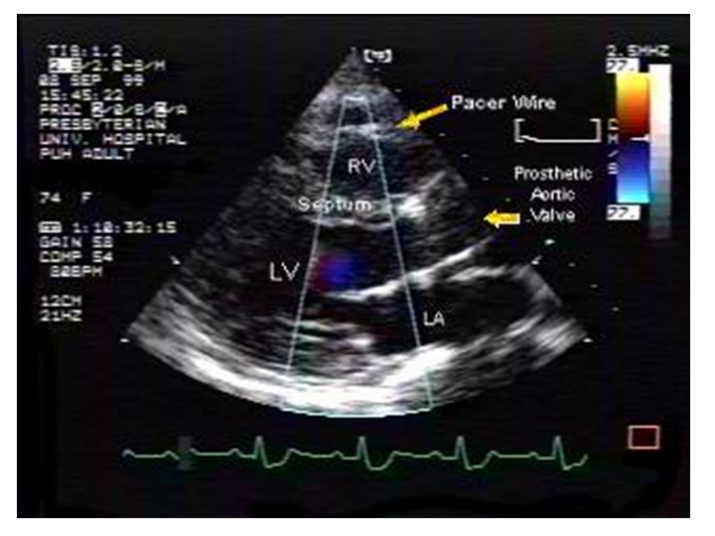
Parasternal long axis view showing an abnormal color flow signal arising from a thick interventricular septum with a predominant flow away from the transducer into the left ventricular cavity. A predominant diastolic component is shown. The position of the pacer wire and prosthetic aortic valve are also shown. (RV = right ventricle, LV = left ventricle, LA = left atria).
